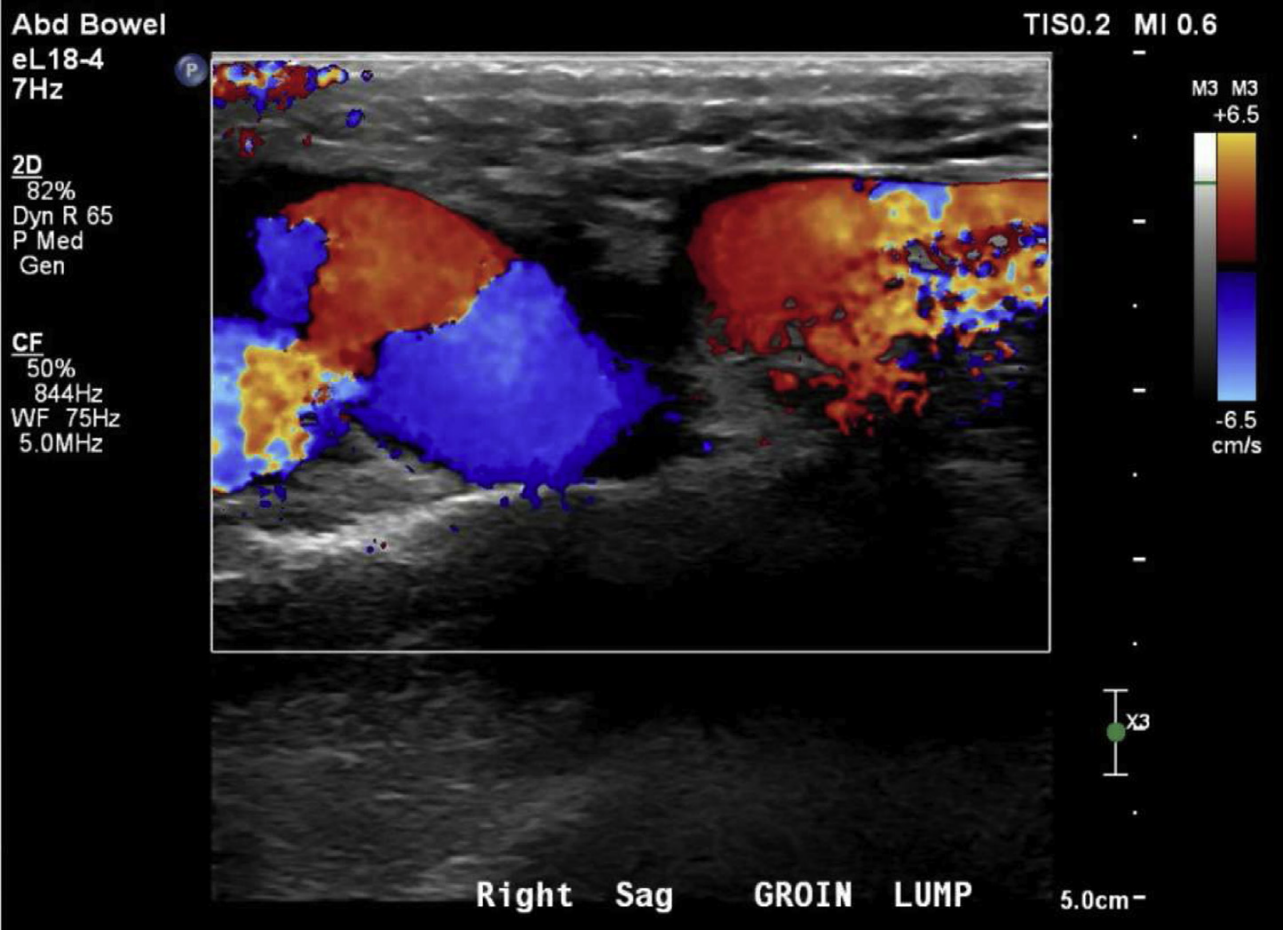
Color duplex ultrasound image of right saphenous femoral junction showing large, varicose femorofemoral collateral with right to left shunting of venous blood.
